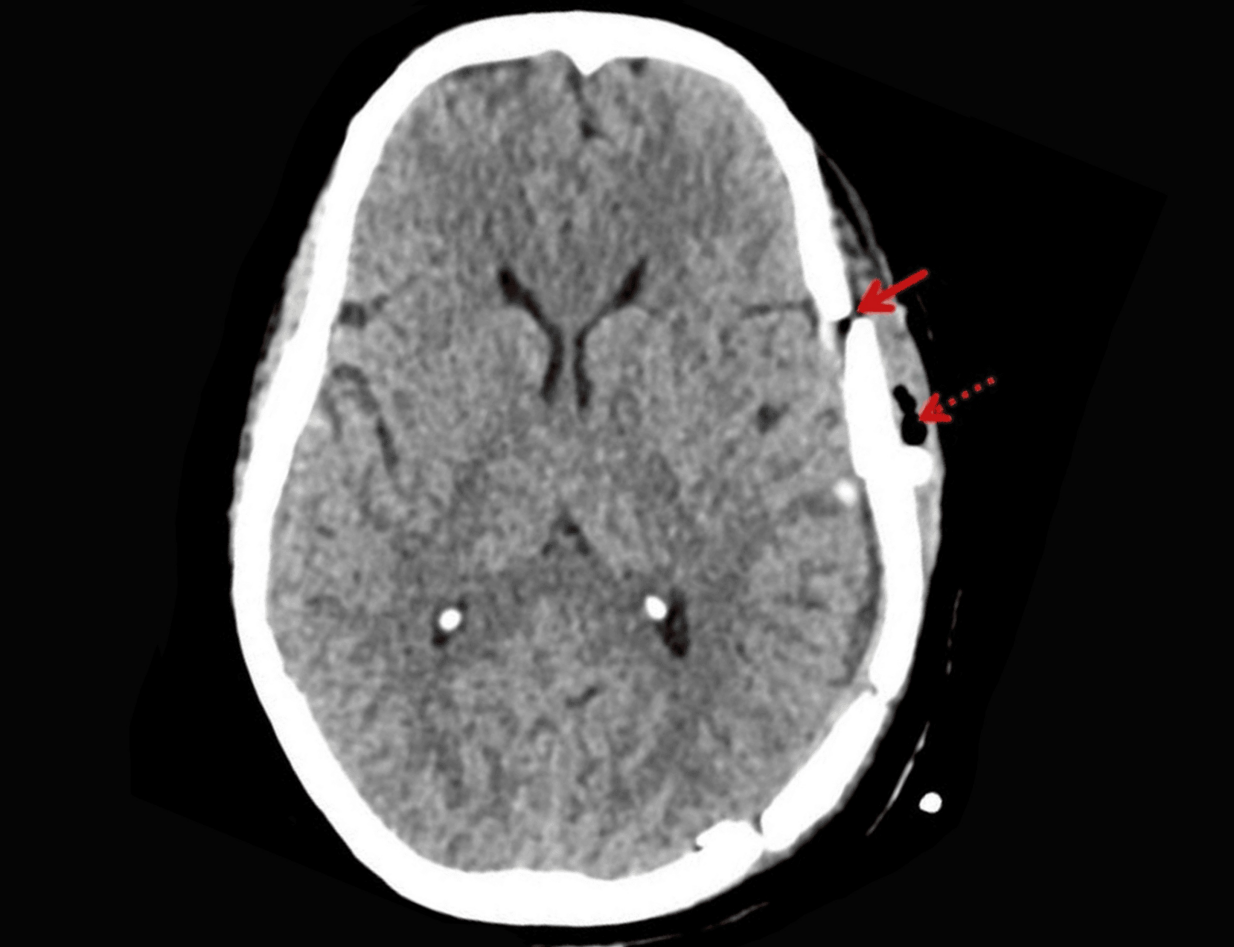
Postoperative NCCT showing complete resolution of EDH with cranioplasty defect (arrow) and subcutaneous emphysema (dotted arrow). NCCT: non-contrast computed tomography; EDH: extradural hematoma
